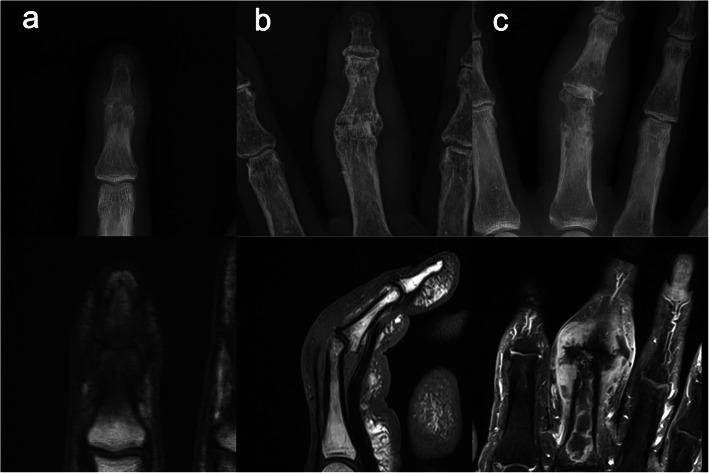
a The right index finger of a 35-year-old man. The distal interphalangeal joint shows joint space narrowing in a simple radiograph, while T1 weighted image shows intramedullary marked low signal intensity, indicating osteomyelitis. b The right long finger of an 80-year-old woman. The proximal interphalangeal joint shows joint space narrowing and radial angulation deformity in a simple radiograph; however, a normal marrow signal is shown in the T1 weighted image. c The right long finger of a 58-year-old woman. The proximal interphalangeal joint shows definite osteolysis in a simple radiograph. The proximal phalanx reveals extensive intraosseous abscess in contrast-enhanced T1 weighted image
